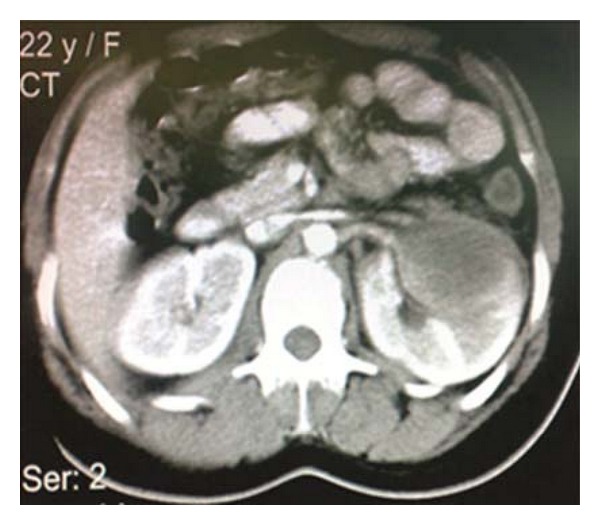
CT scan shows hypodense renal mass with ring enhancement.
